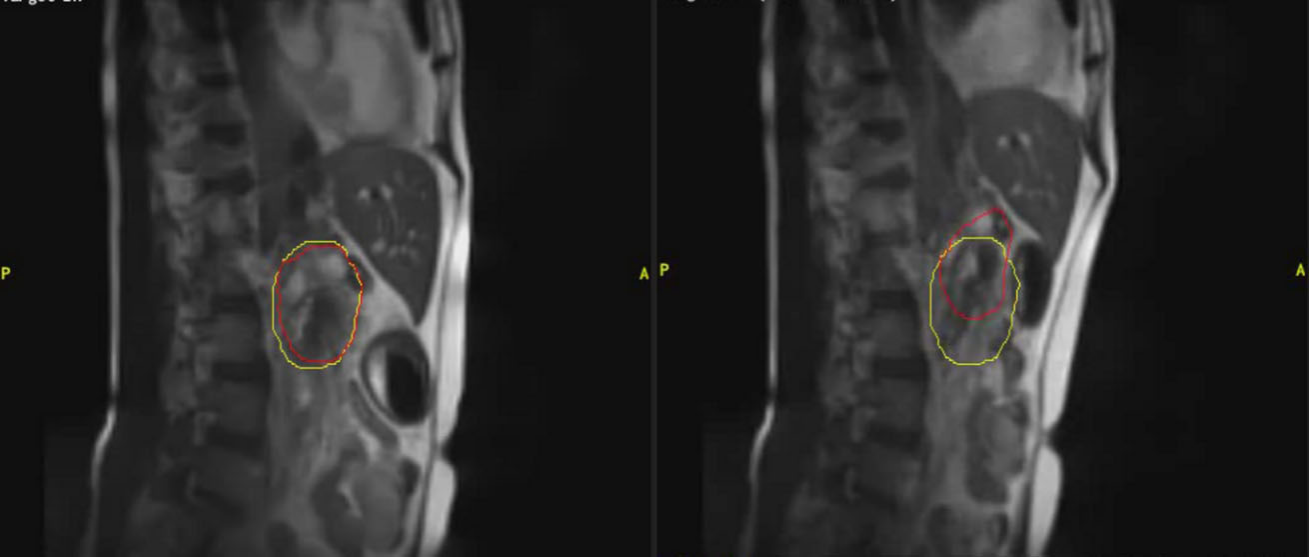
An example of clinical MRI-Linac images utilized for intra-fractional management. The panel on the left shows a patient receiving pancreatic radiotherapy while imaged with a deep inspiratory breathe hold. The panel of the right shows the same patient breathing freely. The red contour depicts the tracking contour and the yellow contour depicts the treatment envelope boundary.
